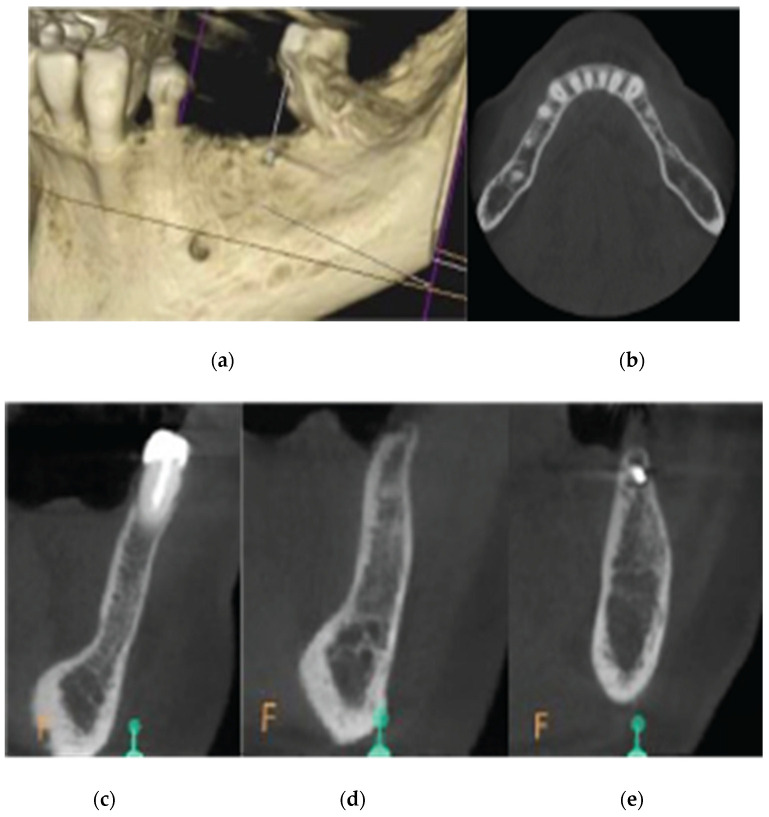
Pre-op CBCT situation—(a) 3D representation of the defect, (b) axial mandibular image. (c) Cross-sectional images of site 34, (d) site 35, and (e) site 36.
